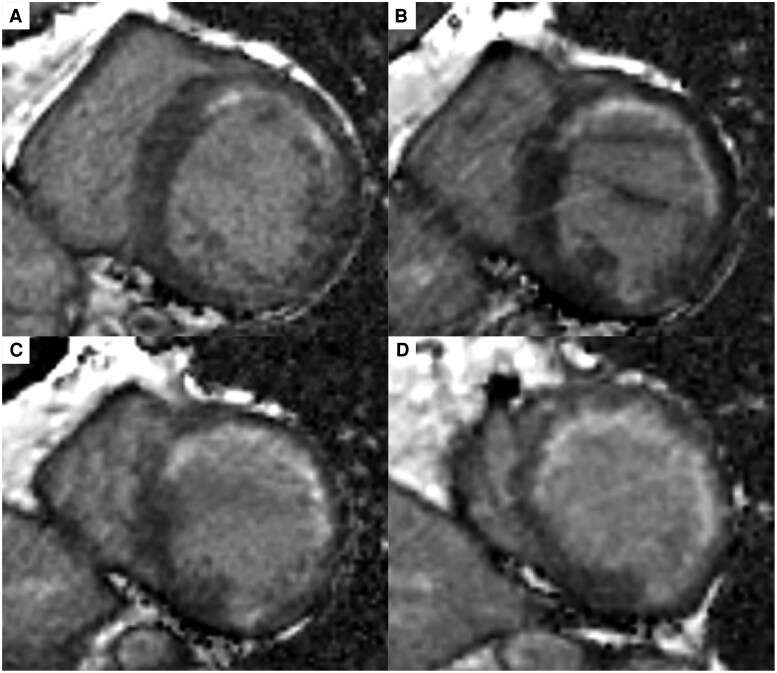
Cardiac magnetic resonance imaging. Subendocardial late gadolinium enhancement was observed in left anterior descending artery territory. Short-axis views of the left ventricle at the basal (*A*), mid-ventricular (*B* and *C*), and apical (*D*) levels.
